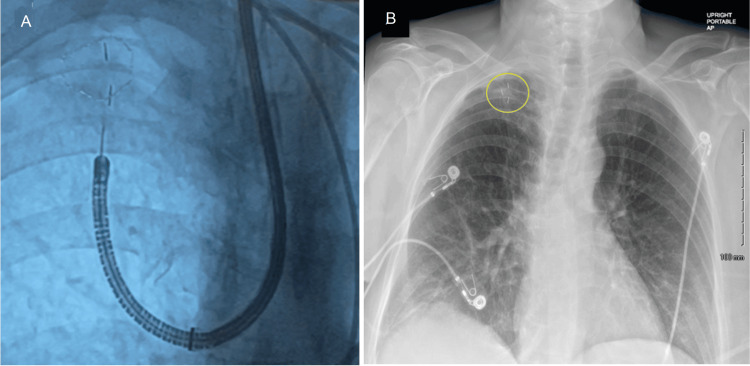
(A) Visicoil gold 5x0.5 mm fiducial markers placed using augmented fluoroscopy; (B) Post-procedural chest radiograph showing three fiducial markers placed in a triangulated pattern around the lesion and no evidence of pneumothorax
